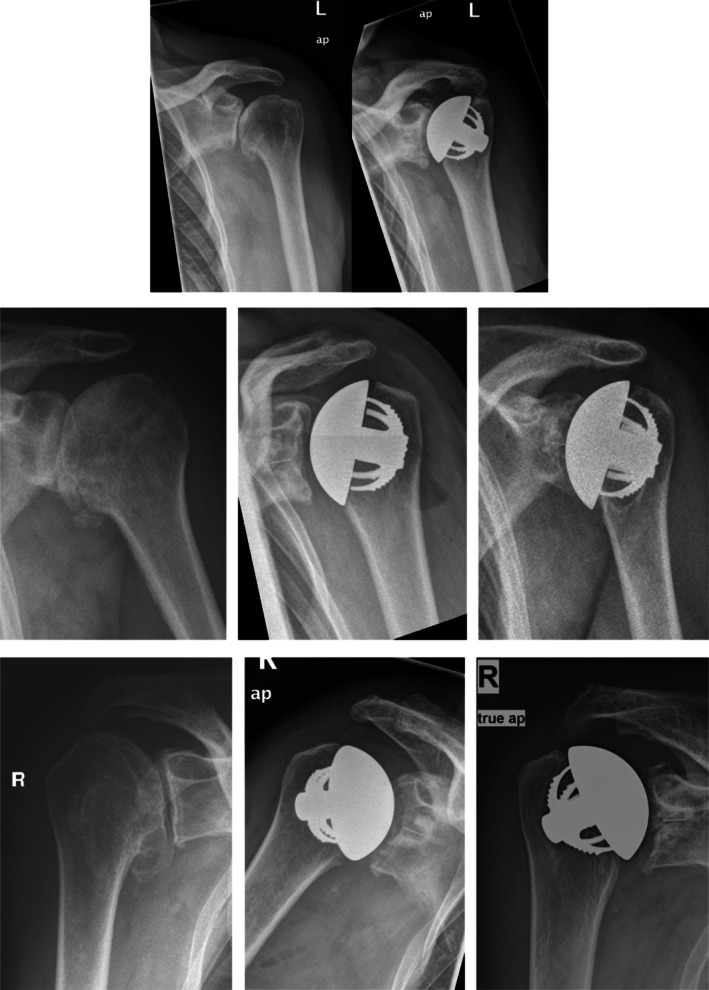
Pre‐ and postoperative radiograph of a left shoulder. Left shoulder of a 68‐year‐old female patient at the time of surgery with primary glenohumeral osteoarthritis. Left: Preoperative. Center: 4 days postoperative. Right: 12 years and 3 months postoperative. Right shoulder of a 61‐year‐old male patient at the time of surgery with primary glenohumeral osteoarthritis. Left: Preoperative. Center: 3 days postoperative. Right: 13 years and 5 months postoperative.
